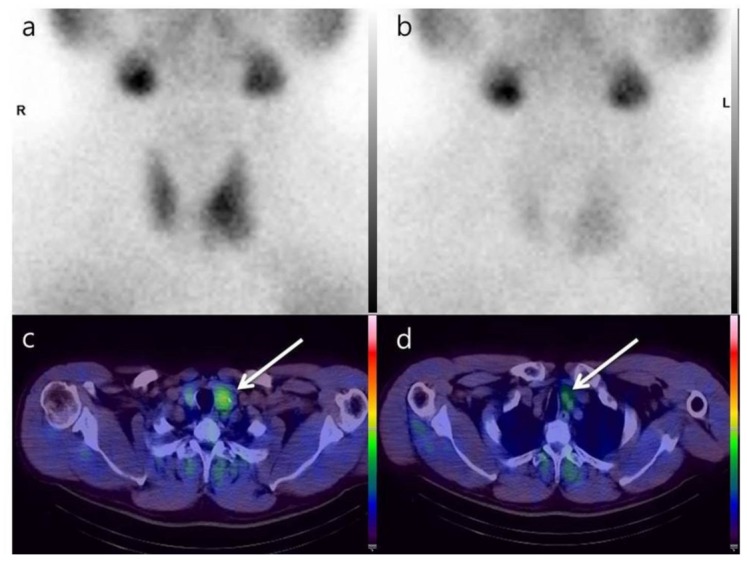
Parathyroid lesions detected by Tc-99m MIBI single photon-emission computed tomography/computed tomography. Two lesions (white arrows) with focal radioactive uptake were detected in the left thyroid lobe (c,d). These lesions were not detected by early (a) or delayed (b) Tc-99m MIBI planar scintigraphy images. R: right; L: left.
